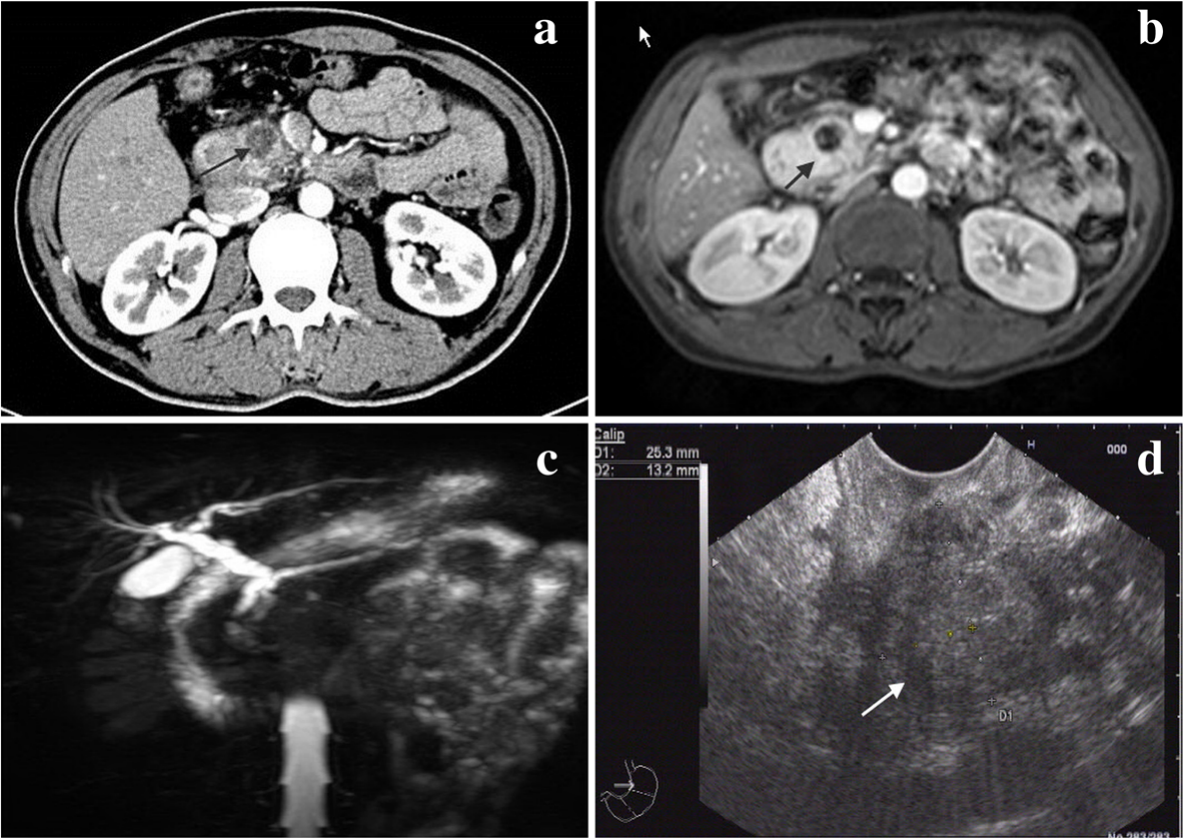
Imaging investigations of the pancreatic head DTF (indicated by the arrow) using (a) abdominal CT scan, (b) MRI scan, (c) MRCP, and (d) EUS.
